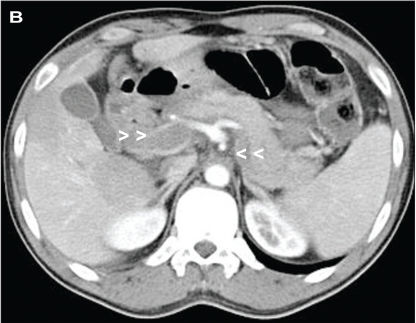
Contrast-enhanced axial computed tomography scan shows portal vein (B, right arrow heads) and splenic veins (C, left arrow heads) in a patient with polycythemia rubra vera and mobile right heart thrombus.
